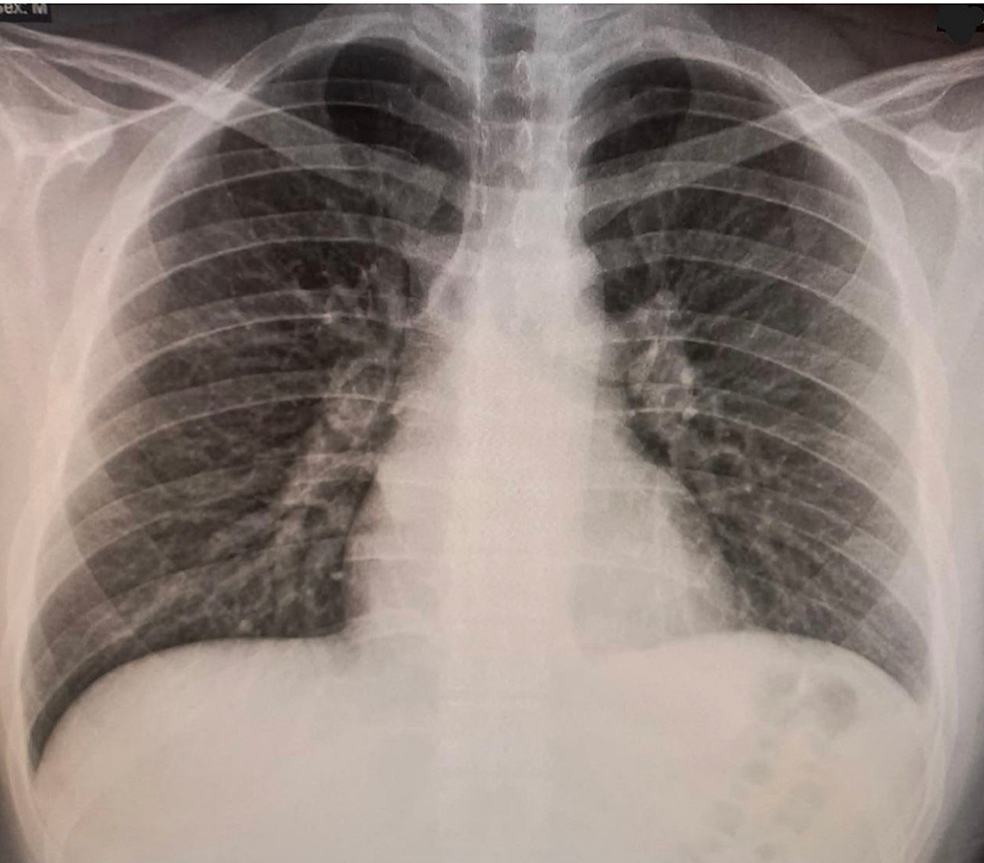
Chest x-ray showing no abnormal findings
